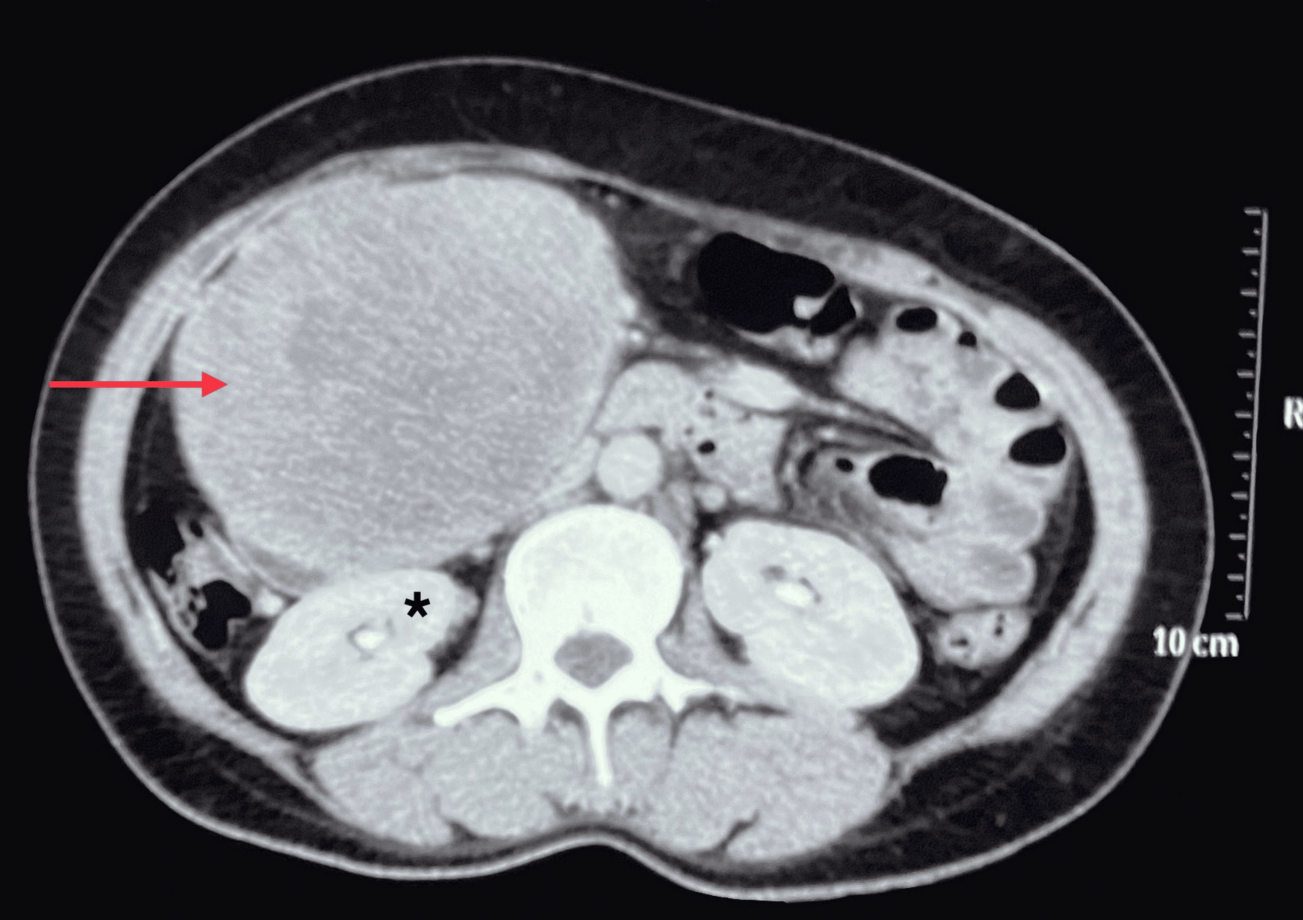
CECT abdomen axial section showing a heterogeneous mass abutting the anterior abdominal wall anteriorly and right kidney posteriorly (asterisk) CECT: Contrast-Enhanced Computed Tomography
